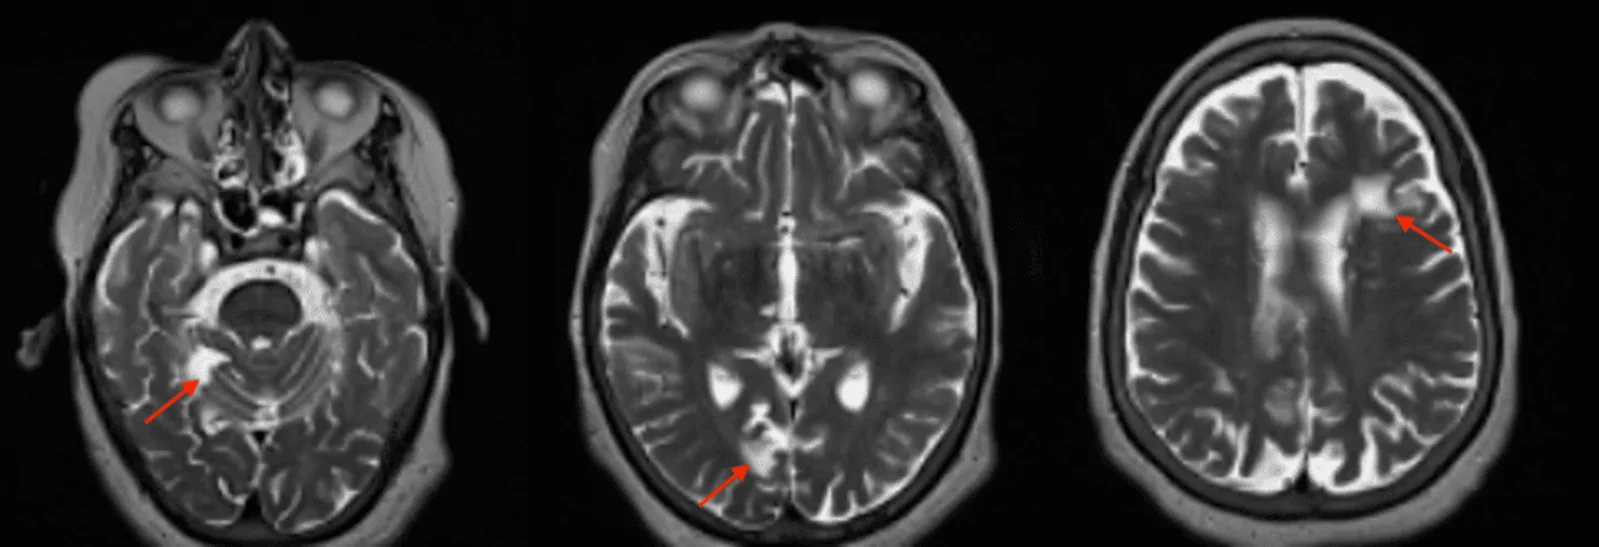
Axial T2‑weighted MRI showing multiple hyperintense lesions (red arrows) in the left frontal, right cerebellar, and right occipital regions. These lesions were consistent with chronic ischemic changes on the basis of the full MRI sequences (FLAIR, T1‑weighted, and DWI) MRI: magnetic resonance imaging, FLAIR: fluid-attenuated inversion recovery, DWI: diffusion-weighted imaging
